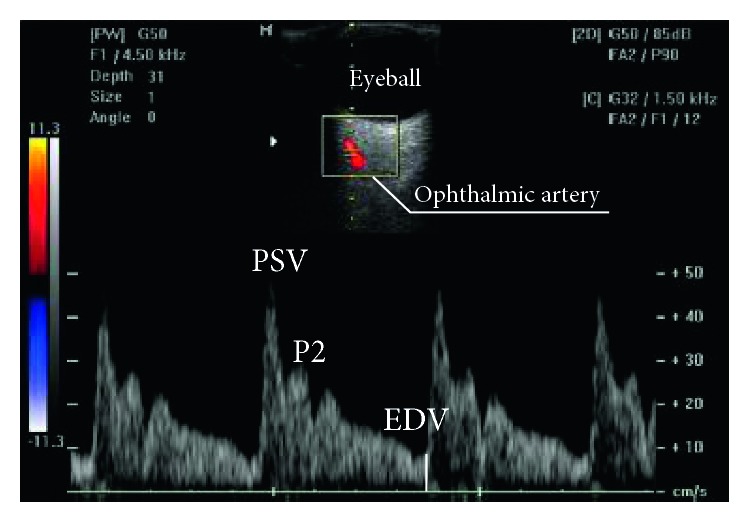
Doppler velocimetry of ophthalmic artery (OA), with identification of the pulse wave velocity (PWV) of the OA. The *X*-axis represents the time (seconds), and the *Y*-axis represents the flow velocity (cm/s). A rapid increase in velocity is observed, with a peak systolic velocity (PSV), followed by a rapid fall, and a new velocity rise with the formation of a second rounded systolic peak (P2), followed by the aortic notch, which closes the systolic cycle. Then, there is an increase in velocity with formation of the diastolic phase of the PWV, which ends with the end-diastolic velocity (EDV). The P2/PSV ratio is referred to as peak ratio [17].
